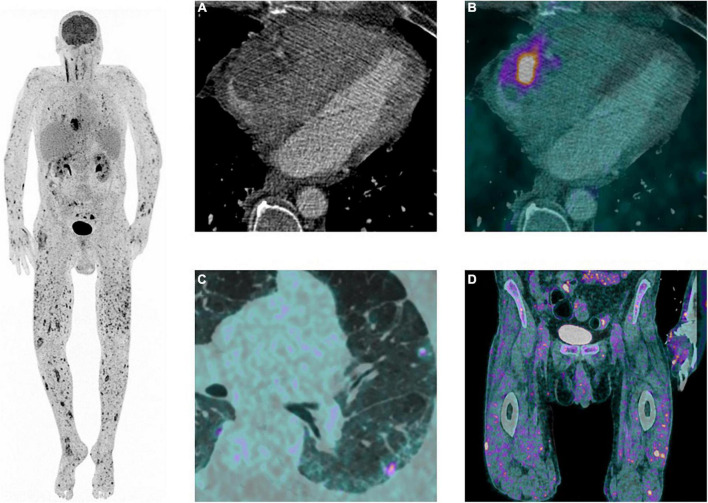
^18^F-fluodeoxyglucose ([^18^F]FDG) Positron emission tomography (PET)/computed tomography angiography (CTA) findings. Left: Maximum intensity projection image of the whole body showing pathological focal diffuse [^18^F]FDG uptake of the trunk as well as the upper and lower extremities. Right: (A) Cardiac CTA showing an heterogeneous mass with irregular margins, in contact with the lateral wall of the right atrium with extension in the pericardial space. (B) Transaxial view of [^18^F]FDG PET/CTA showing pathological [^18^F]FDG accumulation of the right atrial mass, suggestive of an active infectious process. (C) Transaxial view of [^18^F]FDG PET/CTA showing focal subpleural nodules with increase [^18^F]FDG accumulation in the left lung, suggestive of septic emboli. (D) Coronal view of [^18^F]FDG PET/CT showing focal increased cutaneous and subcutaneous [^18^F]FDG accumulation in the proximal part of the lower limbs, suggestive of septic emboli.
